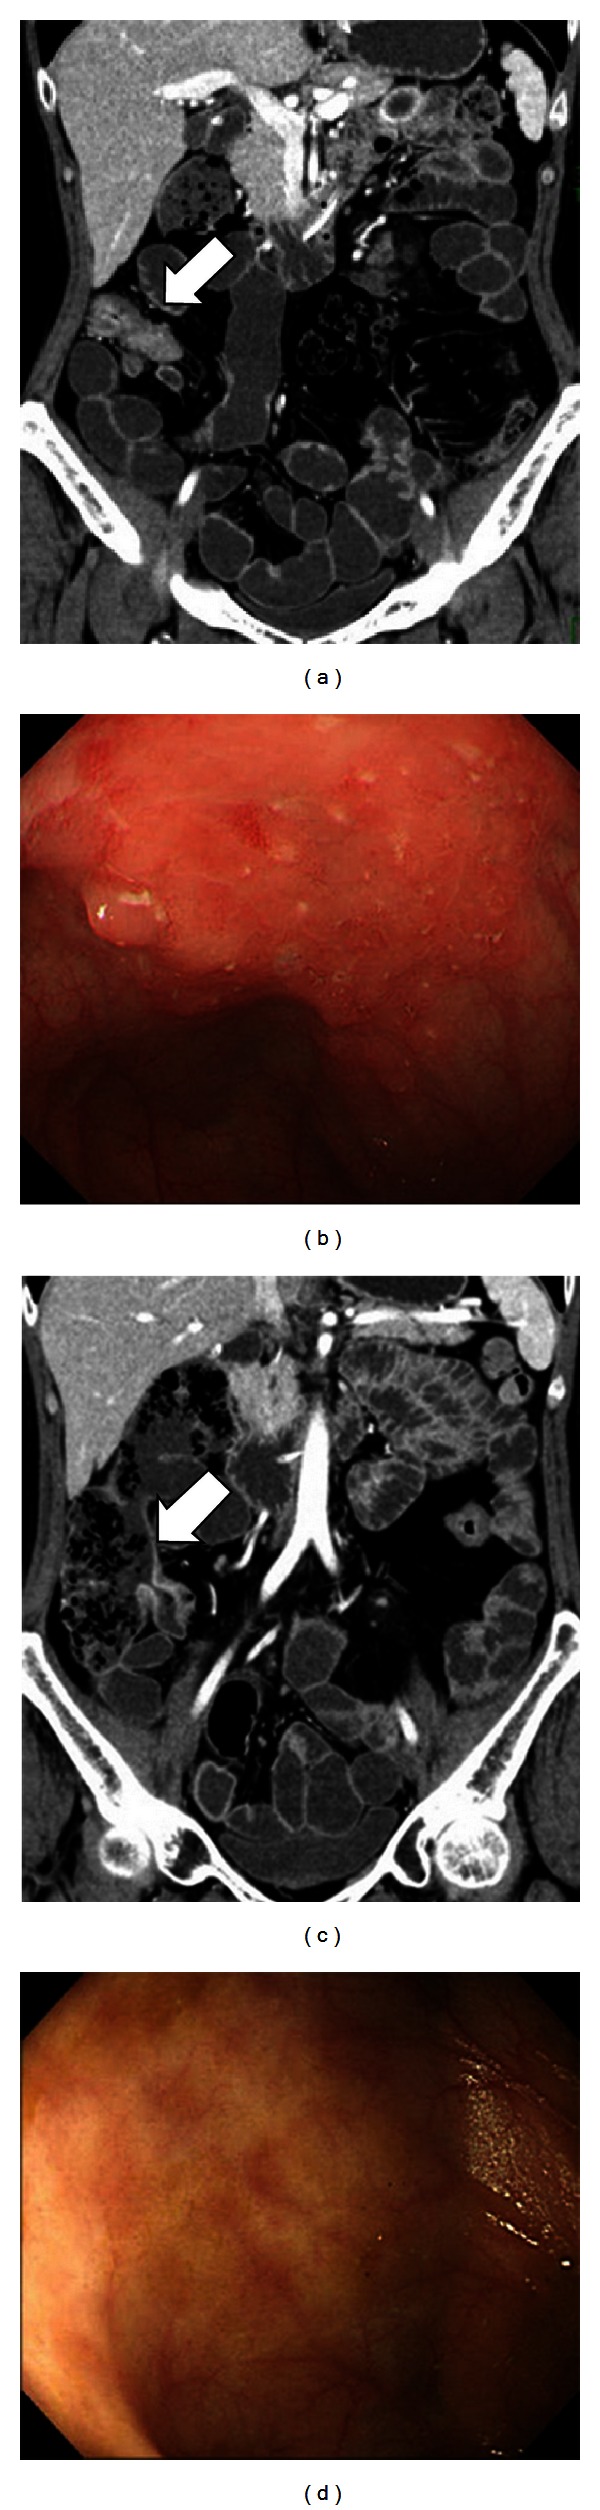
Findings from CT enteroclysis and endoscopic mucosal examination before and after the introduction of biologics. (a) CT enteroclysis before the introduction of biologics. (b) Mucosal findings corresponding to the same location as indicated by the arrow in (a) show edema. (c) CT enteroclysis at 84 days after the first CT enteroclysis showing improvement of the inflammation. (d) Mucosal findings corresponding to the same location as indicated by the arrow in (c) show no edema or ulcer.
